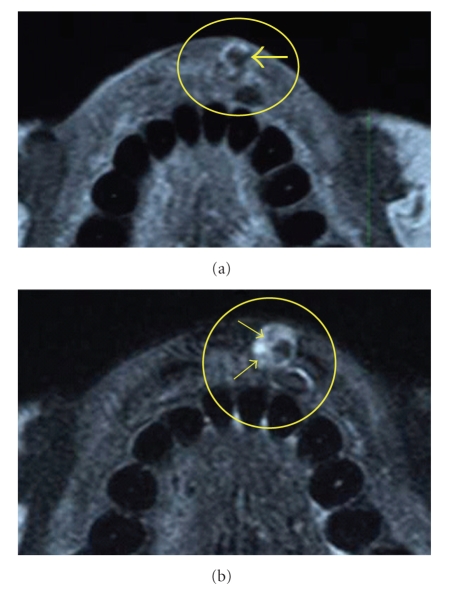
(a) Axial T1-weighed MR image of the lesion (inside yellow circle). This image shows that the margin of the lesion showed an enhanced line with no signal area in the center of the lesion (arrow). TR was 542 (milliseconds) and TE was 9 (milliseconds). The sequence of MR image was FSE, and NEX was 512 × 512. (b) Axial T2-weighed MR image of the lesion (inside yellow circle). This image shows a no signal intensity area in the center of the lesion and also a no signal intensity area beside two low-signal intensity dots (arrows). TR and TE parameters of T2 weighed MR image were 3000 (m sec) and 80 (m sec). The sequence of MR image was FSE, and NEX was 512 × 512.
